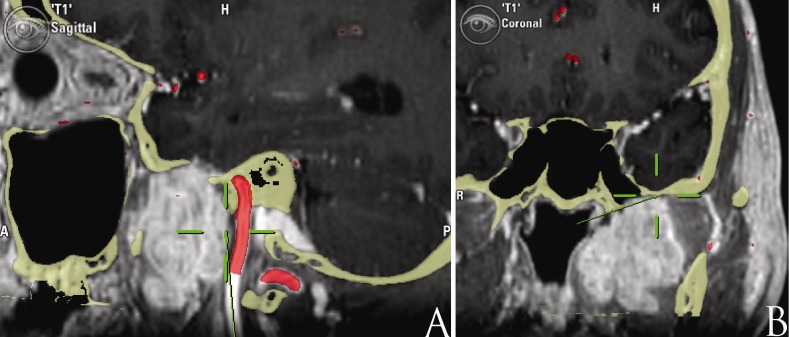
Computed tomography overlay T1-weighted MRI and MRA scan. The arterial vasculature is labeled (red). The image guidance probe (green line) indicates that the carotid artery was accessed and detached from the tumor from the transcervical approach (A) and that foramen ovale was approached and the superior limit of the tumor was accessed through the endoscopic endonasal route (B).
